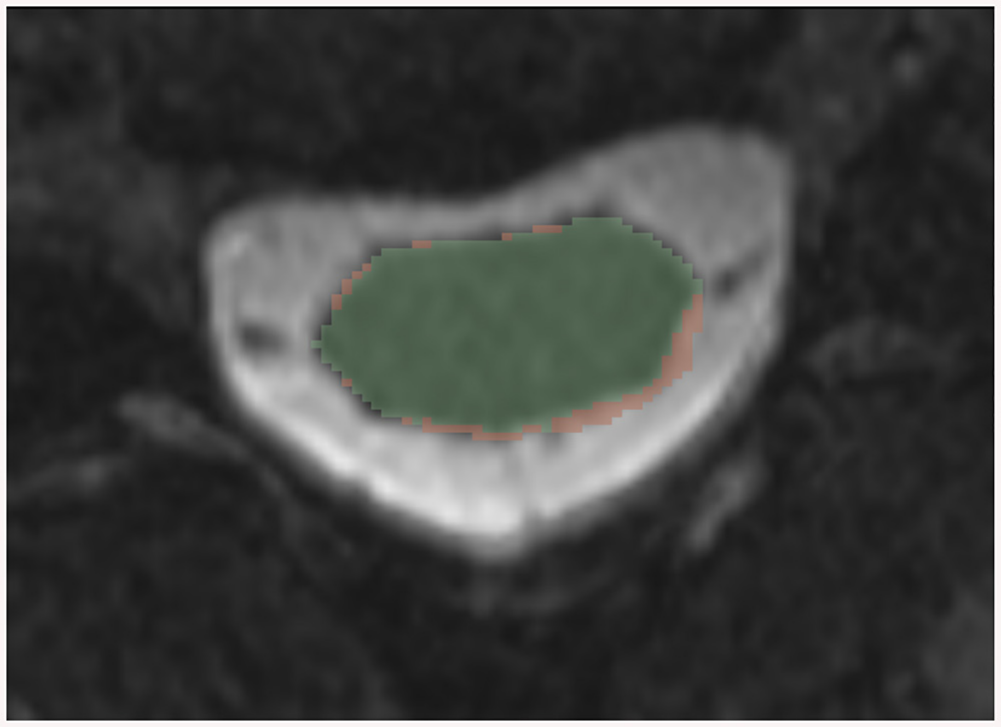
Example of the difference between two raters segmentation of a smoothed T2-weighted MR image. The green region represents the area segmented by Rater 1 and the red region is Rater 2 less Rater 1. Although the segmentations are very similar, and both could subjectively be considered accurate, there is an 8 mm^2^, or 9%, difference in CSA.
